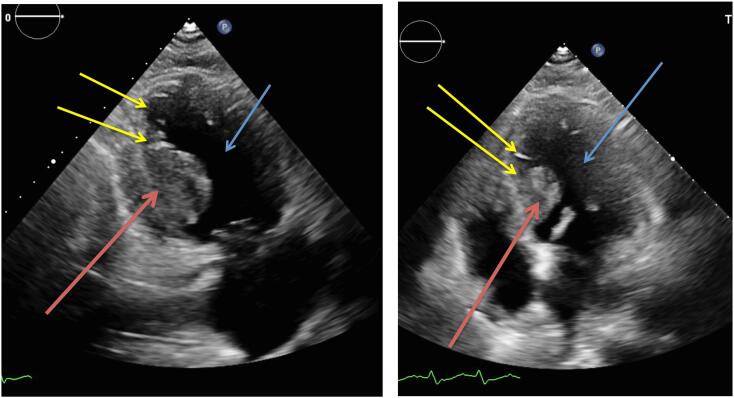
Trans Thoracic Echocardiography (TTE) images showing mass (red arrows) in the left ventricle (blue arrows) at the base of the left ventricular septum (yellow arrows) in the endocardium. (For interpretation of the references to colour in this figure legend, the reader is referred to the web version of this article.)
